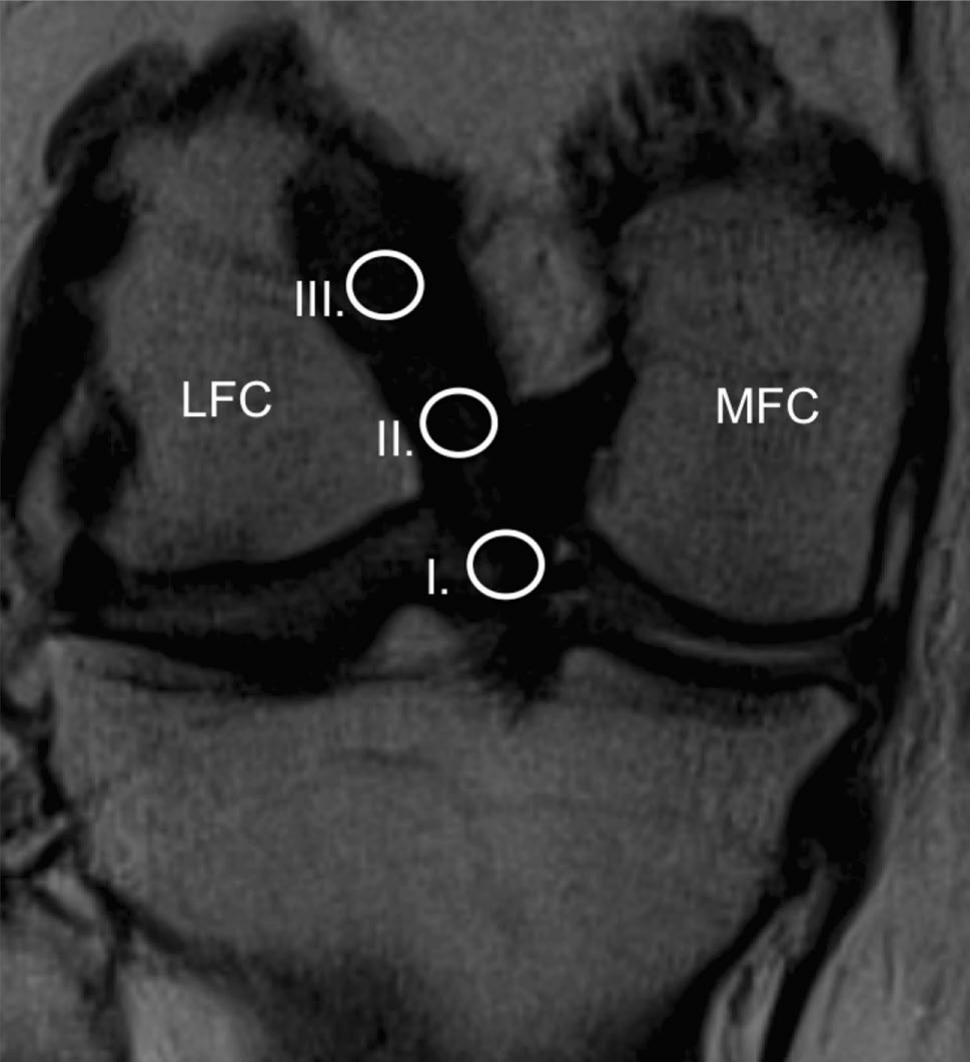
Coronal oblique magnetic resonance imaging scan of a right knee showing the position of the three regions of interest (area of the circle = 0.2 cm^2^), including a (I) distal, (II) middle and (III) proximal site of the ACL. *LFC* lateral femoral condyle, *MFC* medial femoral condyle
